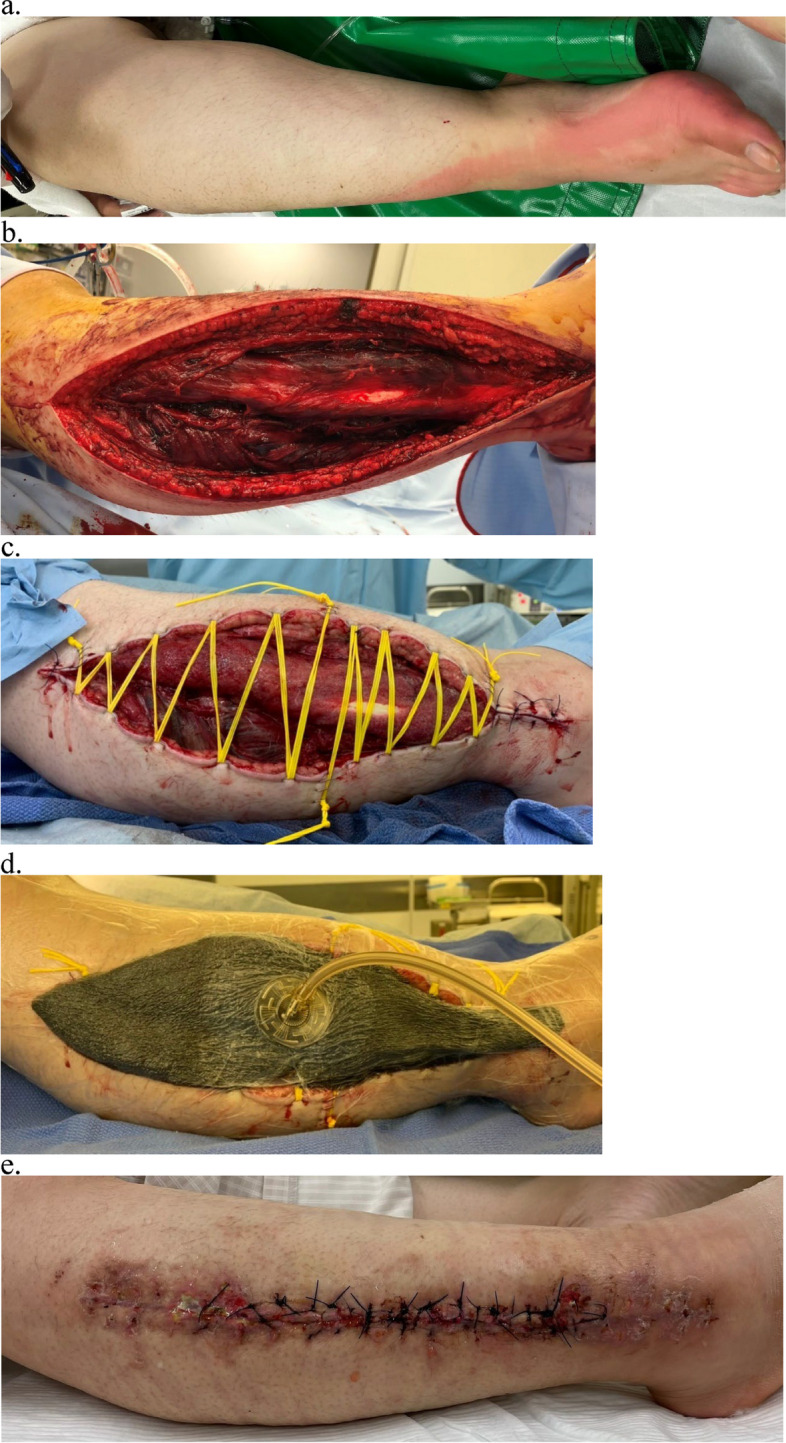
The patient, a 46-year-old female, had taken an overdose of sleeping pills and had lain in the same position for a long time, resulting in compression of the right lower leg (a). A right lower leg X-ray showed no fracture, but there was prominent swelling and pain in the right lower leg at the time of transport. The intramuscular compartment pressure in the right lower leg was elevated to more than 100 mmHg in all four compartments, which led to the diagnosis of compartment syndrome. We immediately performed fasciotomy for the four compartments via a single lateral lower leg incision (b). Forty-eight hours after the fasciotomy, we performed the shoelace technique (c) and placed the NPWT device (d). The shoelace was tightened twice a week, and the wound was completely closed on the 24th hospital day (e). *NPWT* negative pressure wound therapy
